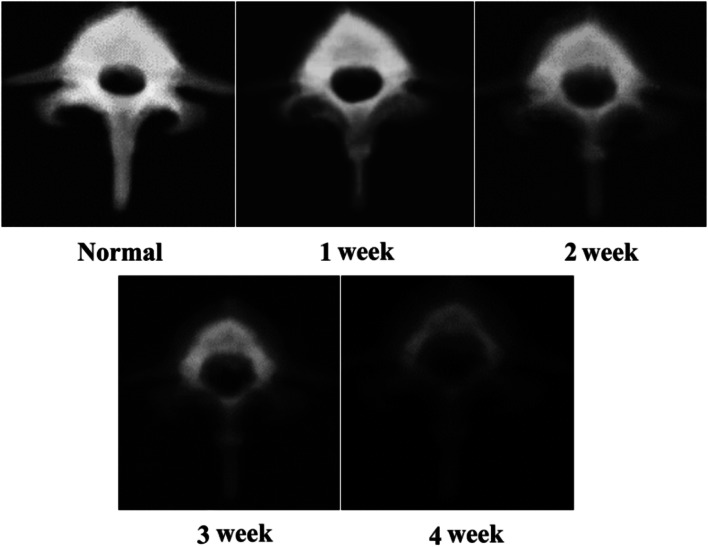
The vertebrae underwent X-ray imaging every week during the decalcification process. Progressive radiolucency over the vertebral body and pedicle was observed during the decalcification process from the first week to the 4th week.
